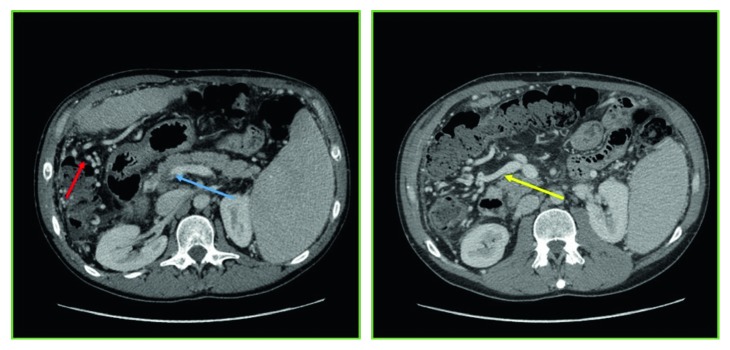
Preoperative CT scan showing PV thrombosis and portosystemic shunts. Blue arrow: PVT. Red arrow: portosystemic shunts. Yellow arrow: right colic vein.
